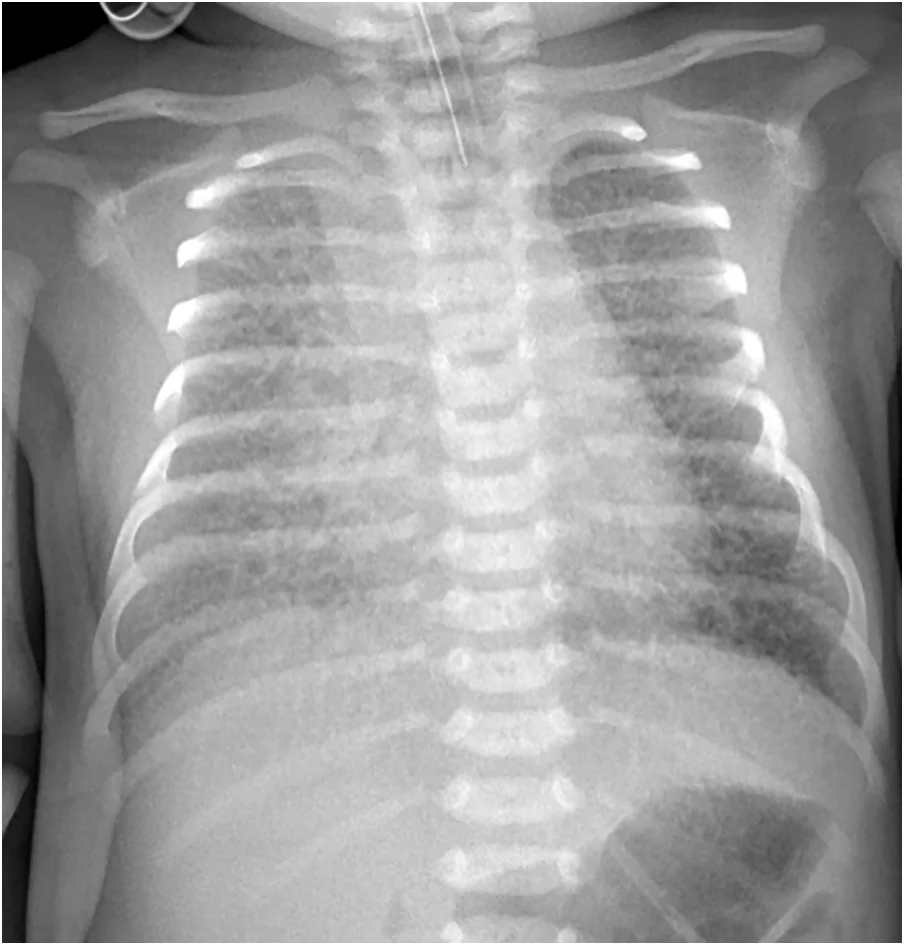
Chest x-ray soon after birth in a neonate with CPVA shows indistinct vascular markings, reduced pulmonary translucency, and normal cardiac silhouette. *CPVA*, common pulmonary vein atresia.
